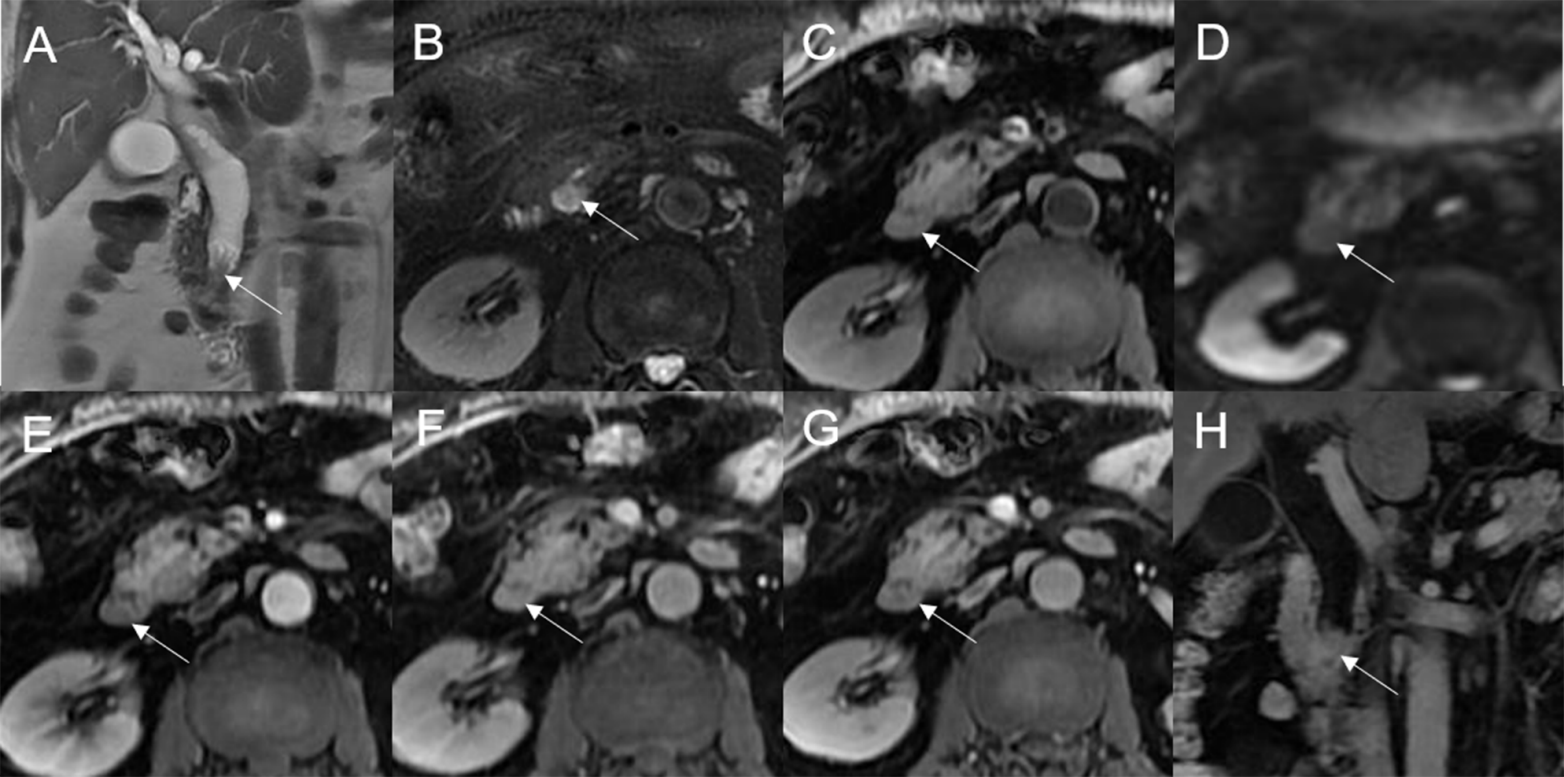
Adenoma in the distal common bile duct. The bile duct casting mass with a frond-like superficial appearance (white arrow) shows hyperintensity on T2WI (A, B), isointensity on T1WI (C), and hyperintensity on DWI (D). There is narrowing of the bile duct lumen at the lesion site and dilation of the duct upstream of the lesion (A). The T1-postcontrast arterial phase (E) shows slight enhancement. The T1-postcontrast portal phase (F) and T1-postcontrast delayed phase (G, H) show moderate enhancement.
